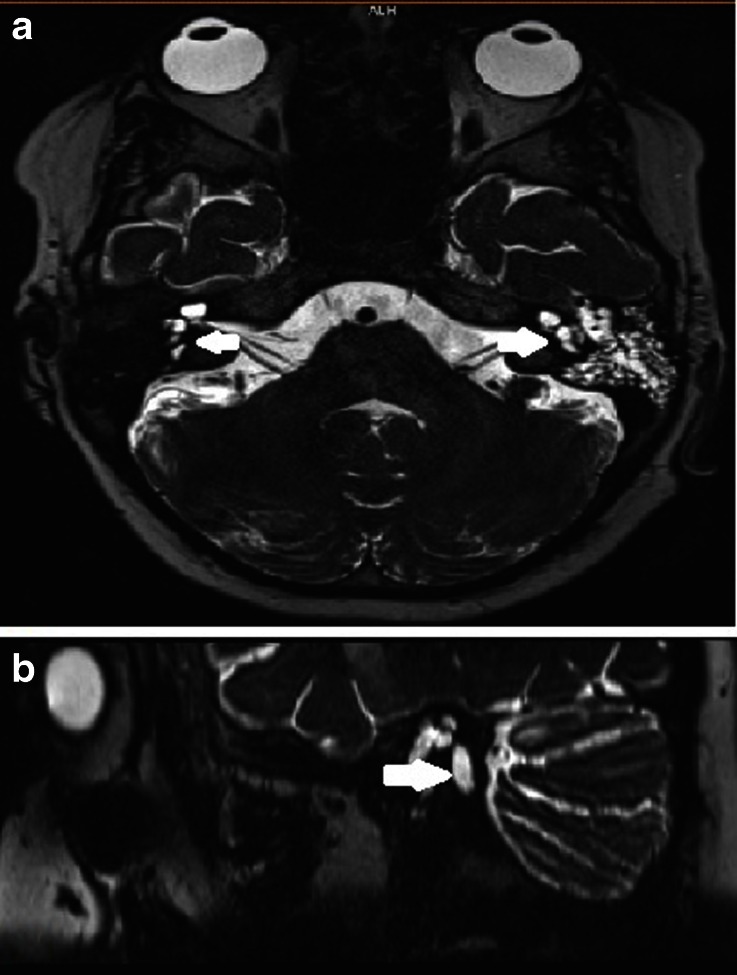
a. MRI scan, axial view, T2 weight shows absence of the apical turns and preserved basal turn of the cochlea (black arrow) and enlarged vestibule(white arrow); b. MRI scan, sagital view shows enlarged vestibular aqueduct (white arrow)
